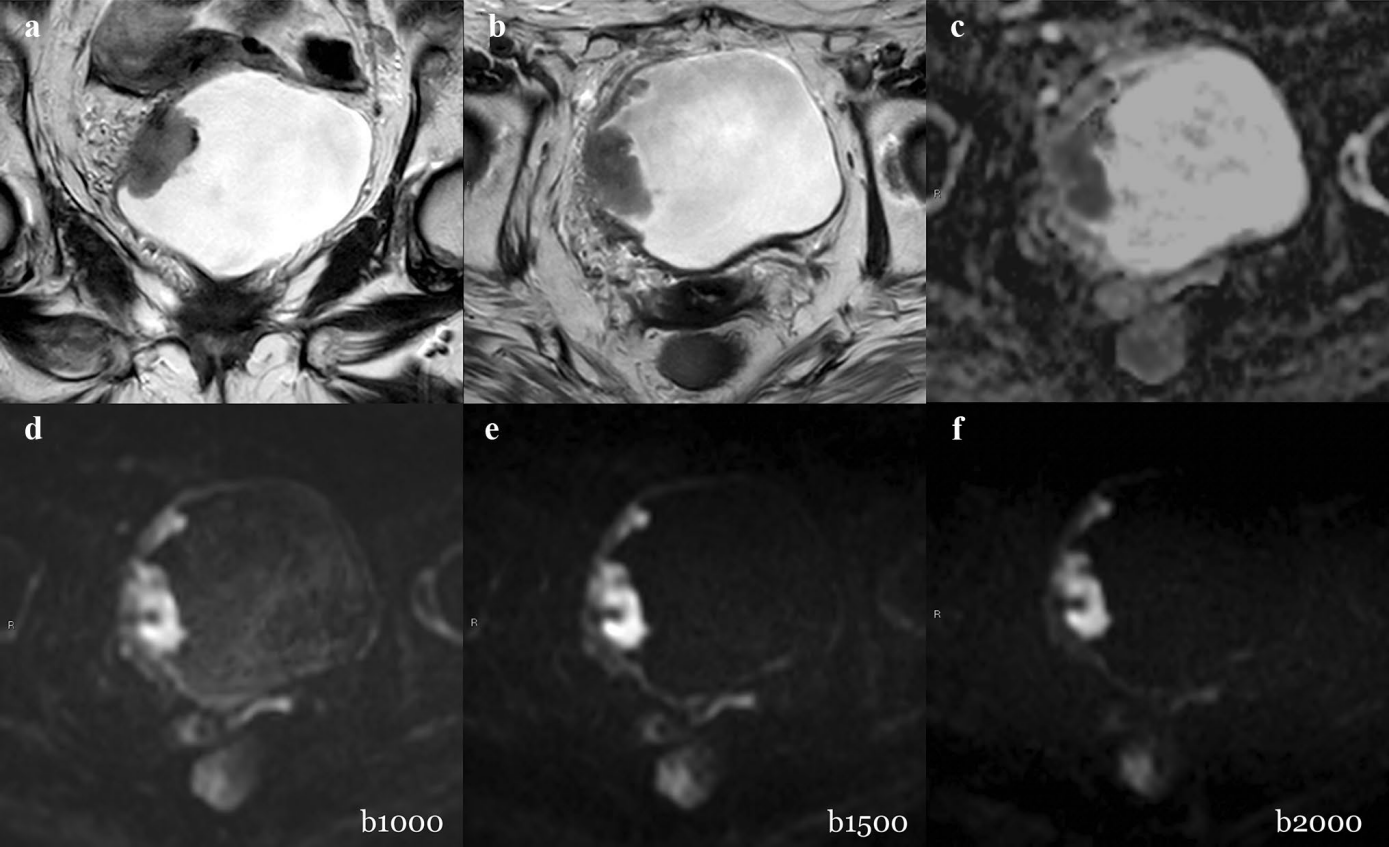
Example of muscle-invasive BC classified correctly. A 79-year-old woman with hematuria and a bladder mass reported after flexible cystoscopy underwent bladder MRI before primary TURB. Coronal (a) and axial (b) T2W imaging showed a thickened right-lateral wall. All gradient strengths (b1000, b1500, and b2000) and ADC map (c) confirmed the restricted diffusion of the tumor extending to the muscular layer. The tumor conspicuity on b1000 (d) was higher than b1500 (e) and b2000 (f) due to better tumor–wall interface visualization. The T stage after TURB was HG-T2 (TURB). DWI = diffusion-weighted imaging; HG = high grade; MRI = magnetic resonance imaging; T2W = T2 weighted; TURB = transurethral resection of the bladder; VI-RADS = Vesical Imaging-Reporting and Data System
